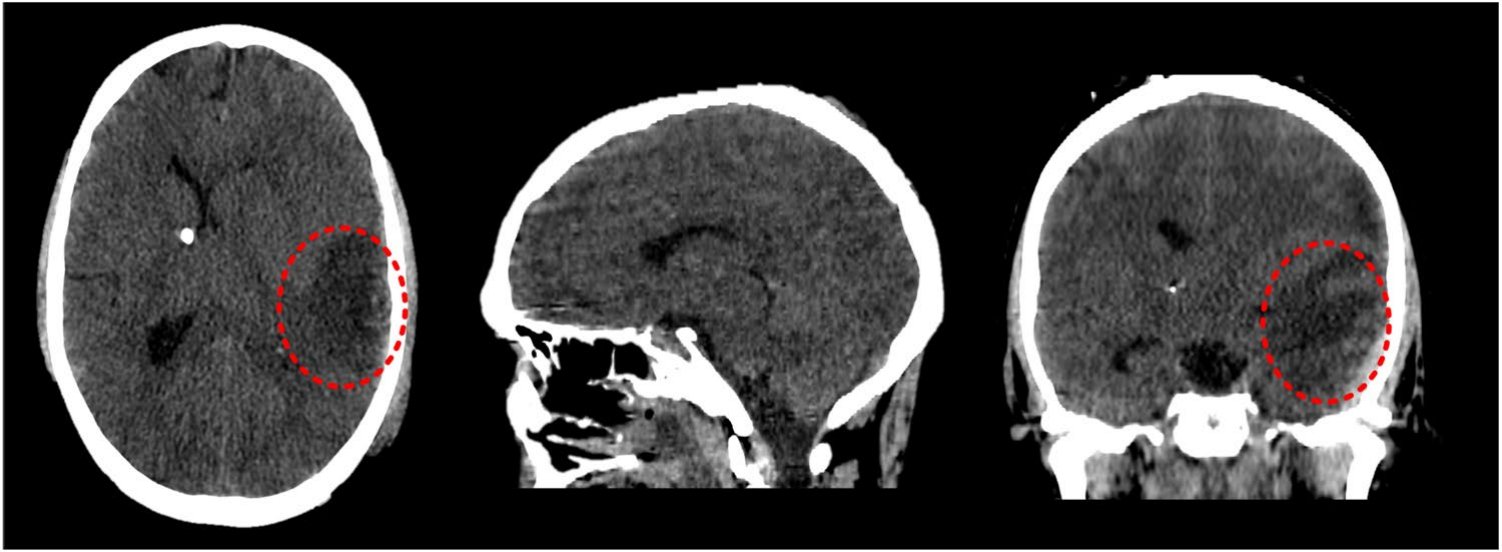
CT images of a patient with localized edema
